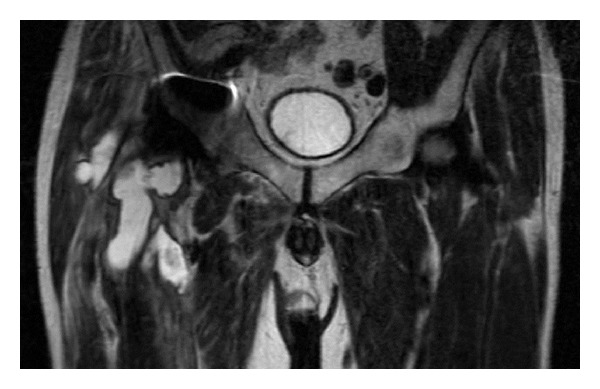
T2-weighted image showing a high intensity lesion with partial low signal intensity, which extends from the lateral aspect of the greater trochanter to the anterior intermuscle of the thigh.
